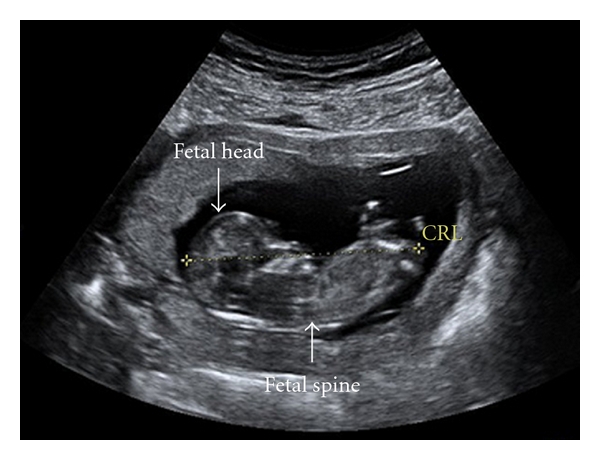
Ultrasound image of a 12-week fetus. The broken yellow line is the crown rump length (CRL) measurement.
